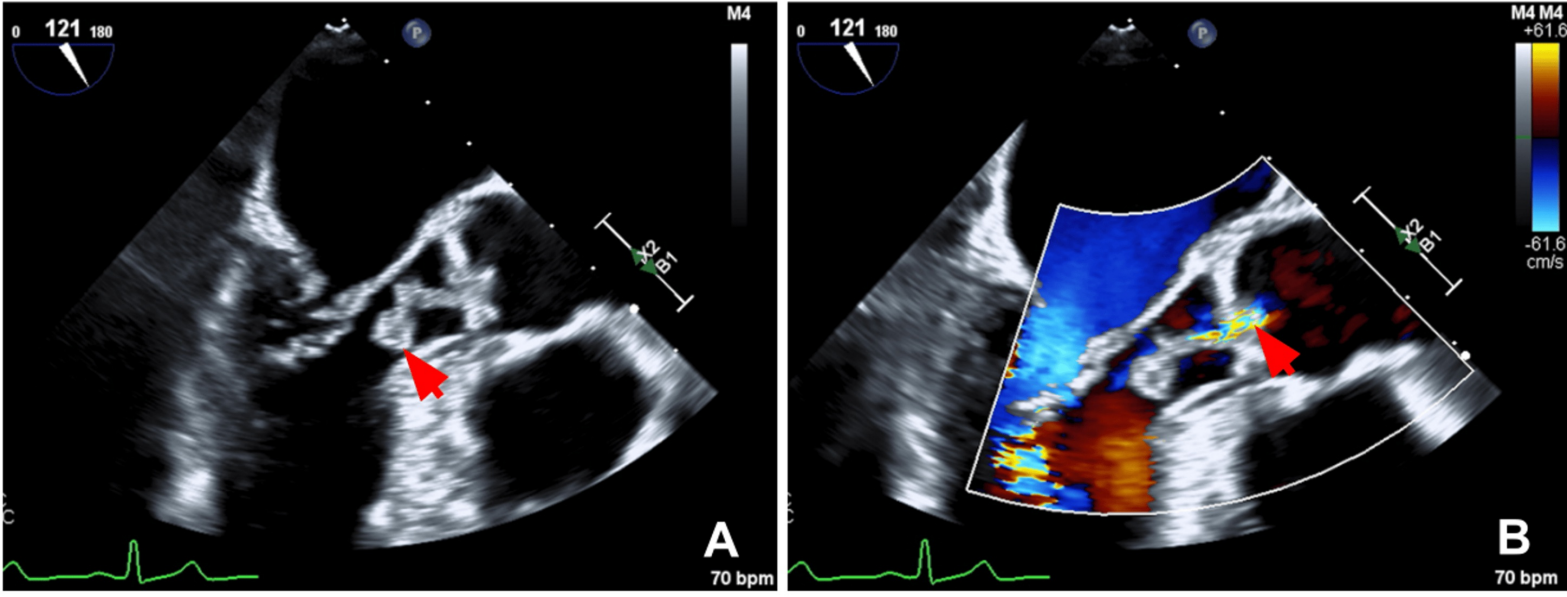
Preoperative transesophageal echocardiography of the aortic valve (A) Long-axis view demonstrating a 1.8-cm mobile mass attached to the aortic valve (red arrow). (B) Color flow Doppler imaging during diastole showing a central jet of mild aortic regurgitation (red arrow).
